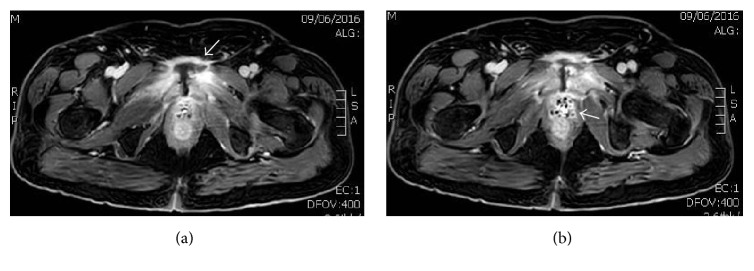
MRI axial imaging of the abdomen and pelvis pre- and postadministration of intravenous Dotarem® contrast. (a) Further distention of the symphysis pubis joint secondary to an increase in size of an abscess within it (arrow), measuring 3.8*∗*1.8 cm, compared to previous measurement of 2.0*∗*1.5 cm. (b) Apparent pocket of air around the prostatic urethra (arrow) likely representing susceptibility artifact from surgical sutures rather than actual gas bubbles.
